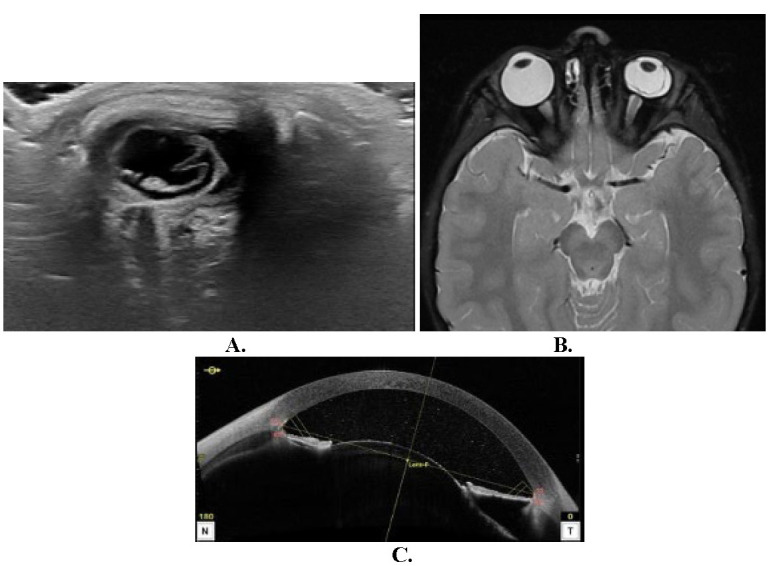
A Orbit ultrasound showing phthisis bulbi and choroid detachment. B. Brain MRI showing phthisis bulbi choroid detachment. C. Anterior OCT revealing choroid detachment
